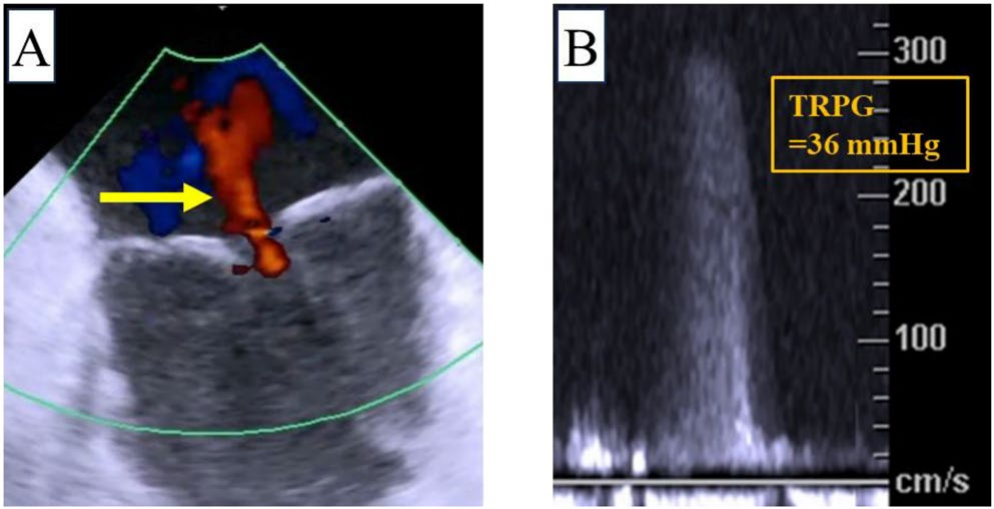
Preoperative assessment of tricuspid valve. (A) Color Doppler flow of tricuspid regurgitation (yellow arrow) in long‐axis tricuspid valve view. (B) Continuous‐wave Doppler across the tricuspid valve. The tricuspid regurgitation pressure gradient was 36 mmHg. TRPG, tricuspid regurgitant pressure gradient.
